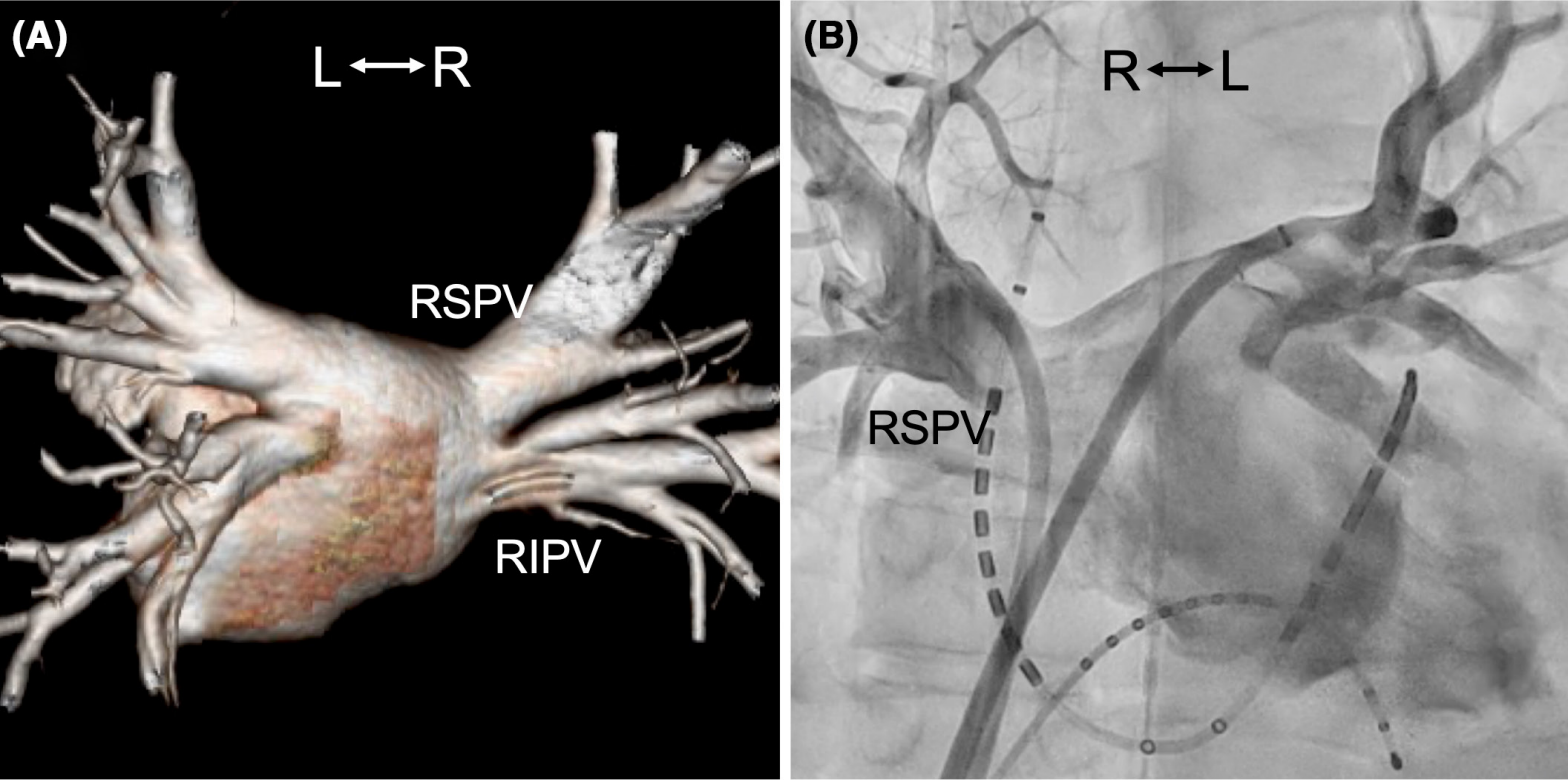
Computed tomography and left atrium angiography before ablation. The computed tomography image (A) and left atrium angiography (B) obtained before ablation demonstrated mild PV stenosis. (PV, pulmonary vein; RSPV, right superior PV; RIPV, right inferior PV).
